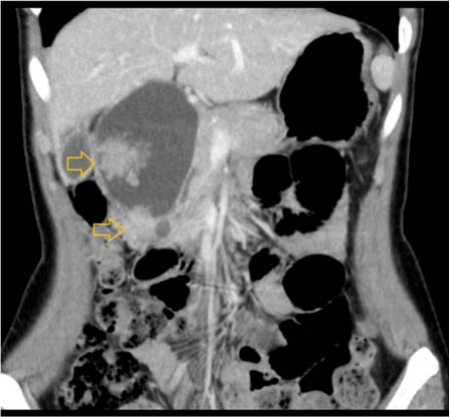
Abdominal CT showed two enhanced tumors (diameter, 4 cm) in the inferior and middle bile duct. *Yellow arrows* indicate the tumor locations
